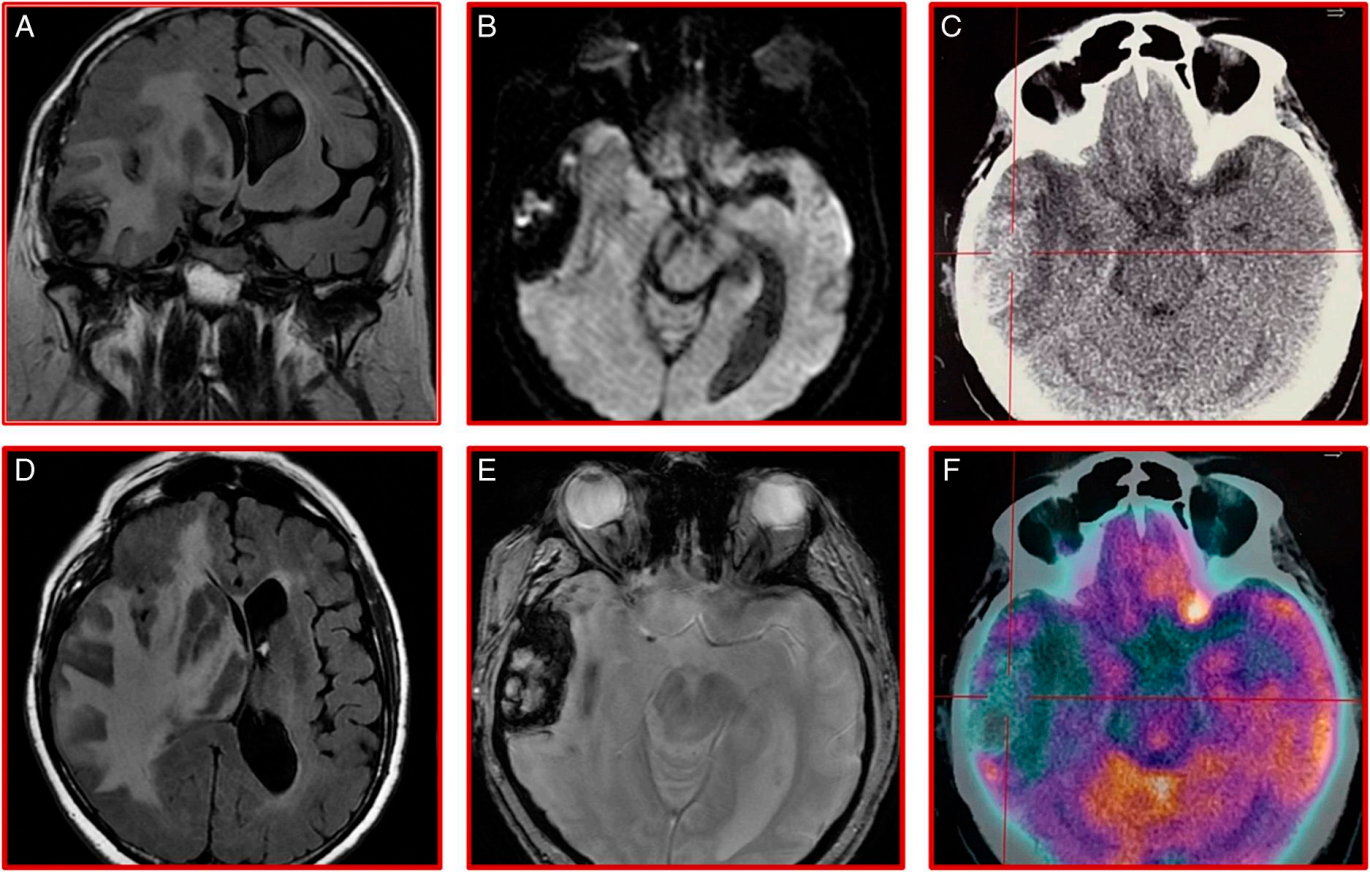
Right temporal radiation necrosis with significant perilesional oedema leading to midline shift. Multimodal neuroimaging of brain radiation necrosis. MRI FLAIR scan: the right temporal mass is shown on the coronal view (A), in which the sellar region appears tumor-free. The extensive perilesional oedema infiltrating the white matter, dissociating the basal nuclei and displacing the midline is also shown on the axial view (D). On axial DWI (B) and T1-weighted Gradient-Echo (E) views, the temporal mass is well-defined but heterogenous with necrotic areas, haemorrhage and hemosiderin deposits. The corresponding large hypometabolic area in the right temporal lobe is shown on 18FDG PET-CT scan (C,F).
